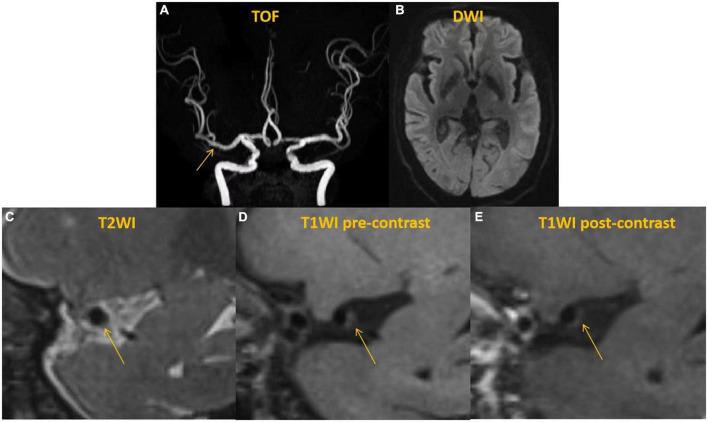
A 62 years-old man without any neuro symptom. Time-of-flight (TOF)-magnetic resonance angiography (MRA) (A) demonstrated a normal appearance. DWI (B) showed no acute infarct with the anterior circulation territory. High-resolution vessel wall MRI (hr-vw MRI) including T2-weighted image (C), pre-contrast T1-weighted image (D) and post-contrast T1-weighted image (E) visualized the plaque on the posterior and inferior side of the right middle cerebral artery (MCA). The plaque showed isointense on T2-weighted image (C) and pre-contrast T1-weighted image (D) and no enhancement on post-contrast T1-weighted image (E).
